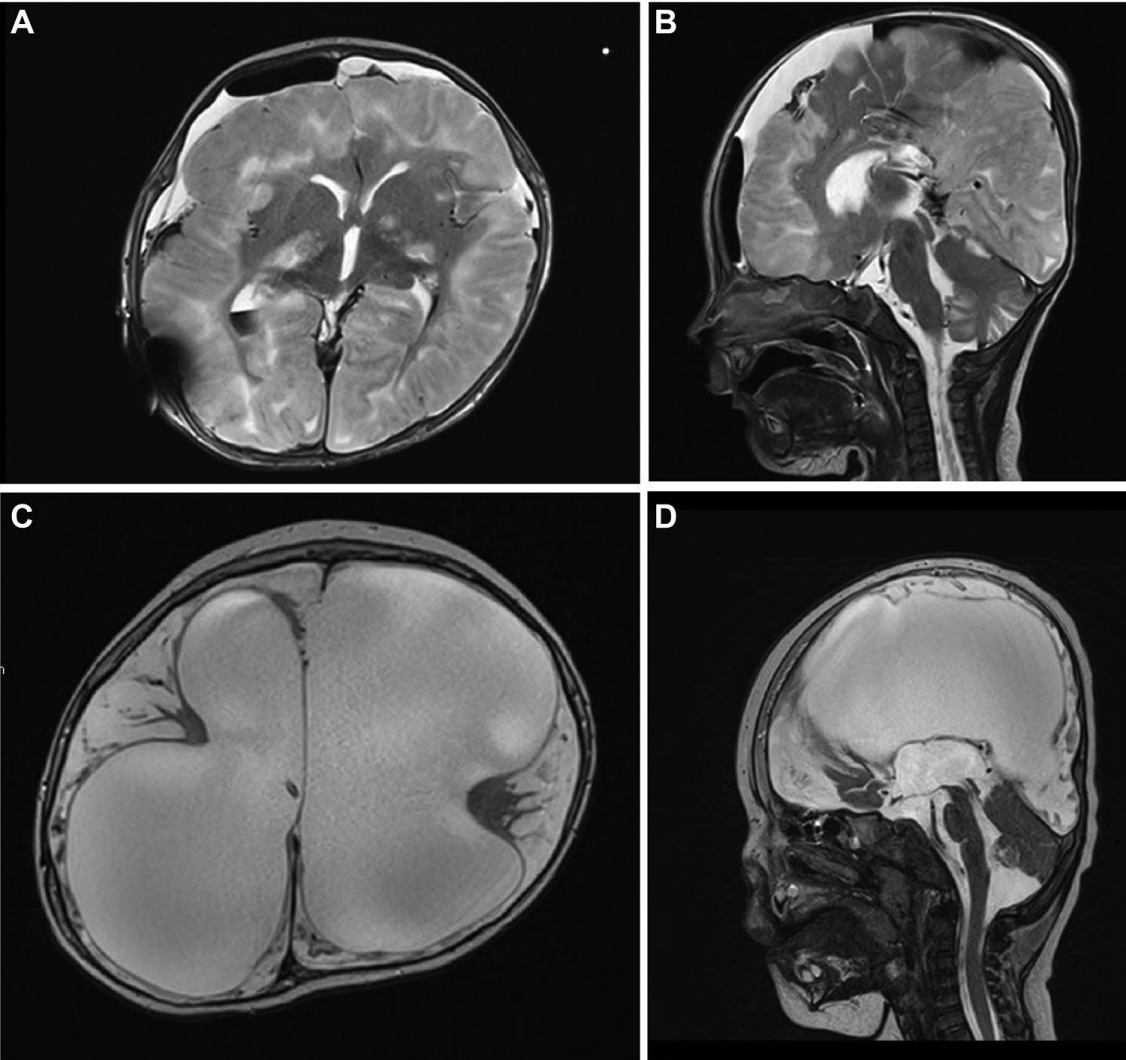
Axial and sagittal T2-weighted MRI sequence showing massive bilateral swelling after hypoxic injury 2 days after the repeated non-accidental trauma (A, B). T2-weighted MRI at follow-up 1 year after the first presentation, showing hydrocephalus ex vacuo because of extensive encephalomalacia after initial bilateral infarction (C, D)
